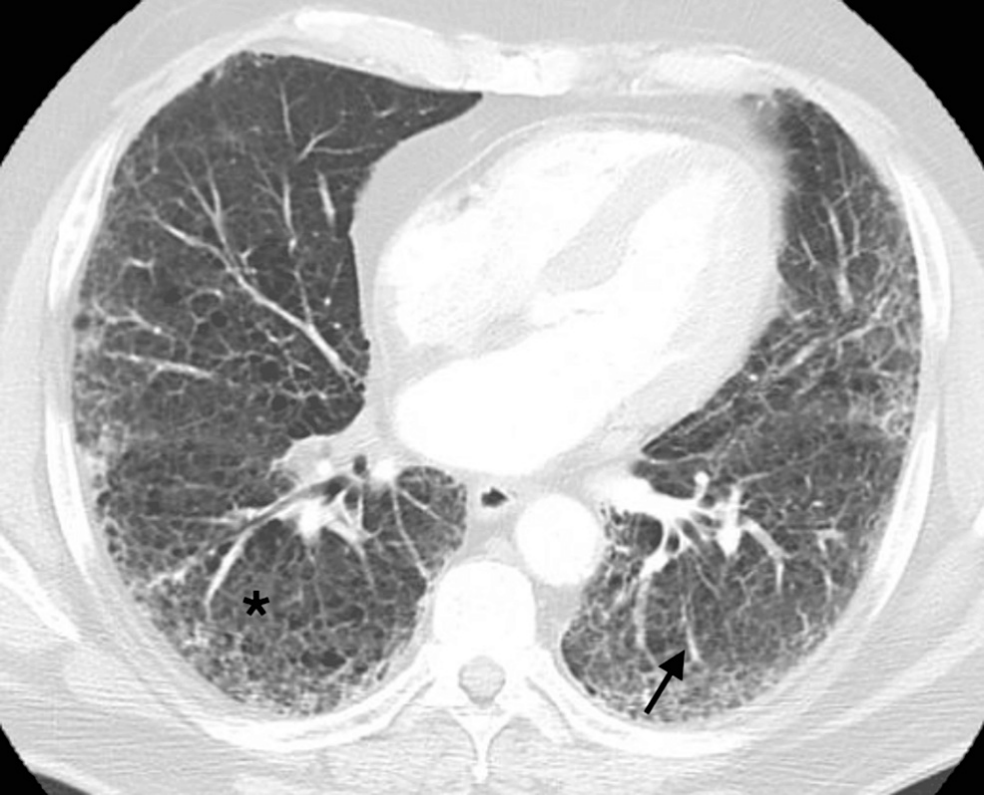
The computed tomography (CT) scan showed diffuse ground-glass alveolar airspace infiltrates (star) and underlying pulmonary fibrosis (arrow)
